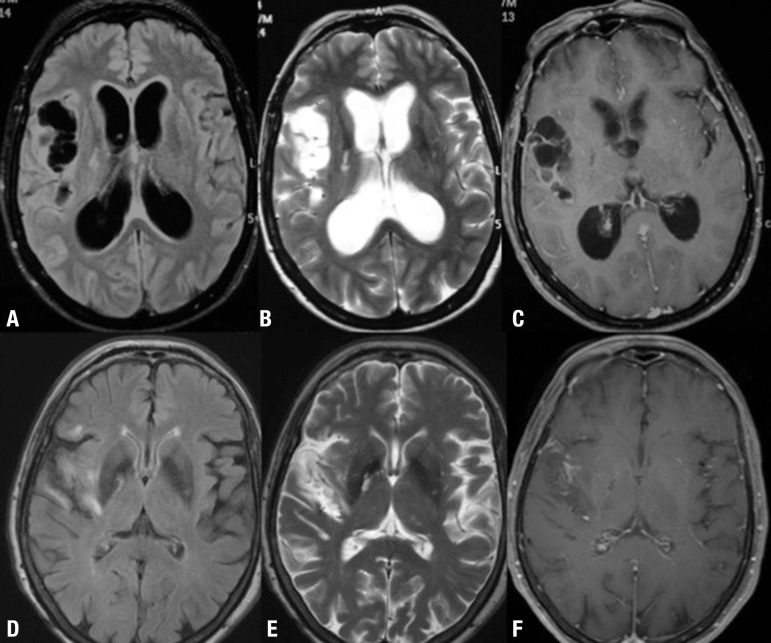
[A] Axial FLAIR, [B] Axial T2WI, [C] Axial T1W1 with gadolinium. [A, B]
